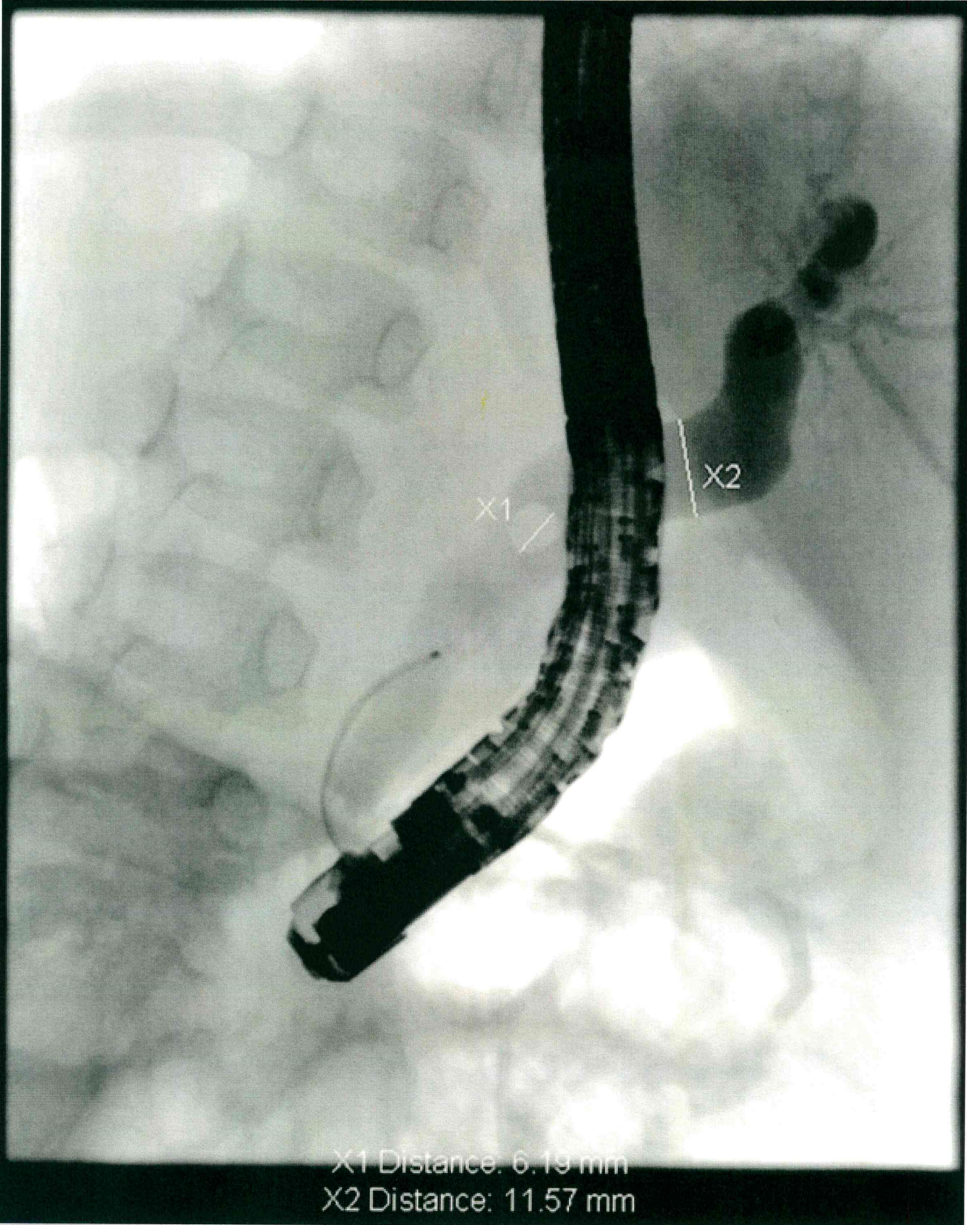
X-ray during ERCP showing choledocholithiasis (X1) and dilatation of the choledochal duct (X2).
